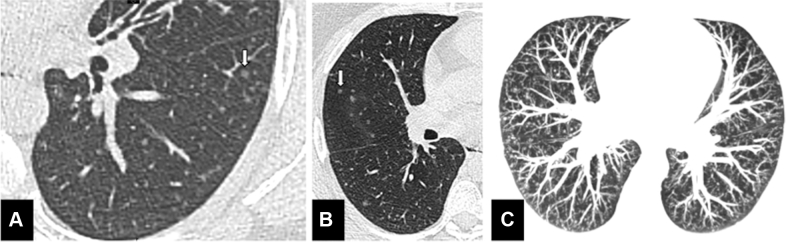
A, B, Axial chest CT scans of the lung window showing multiple diffuse subsolid micronodules without sparing of the right horizontal fissure (B) or left oblique fissure (A). Lesions marked by vertical white arrow demonstrate subtle central lucencie. C, Chest CT scans with maximum intensity projection highlighting the diffuse and randomly distributed lesions throughout both lungs.
